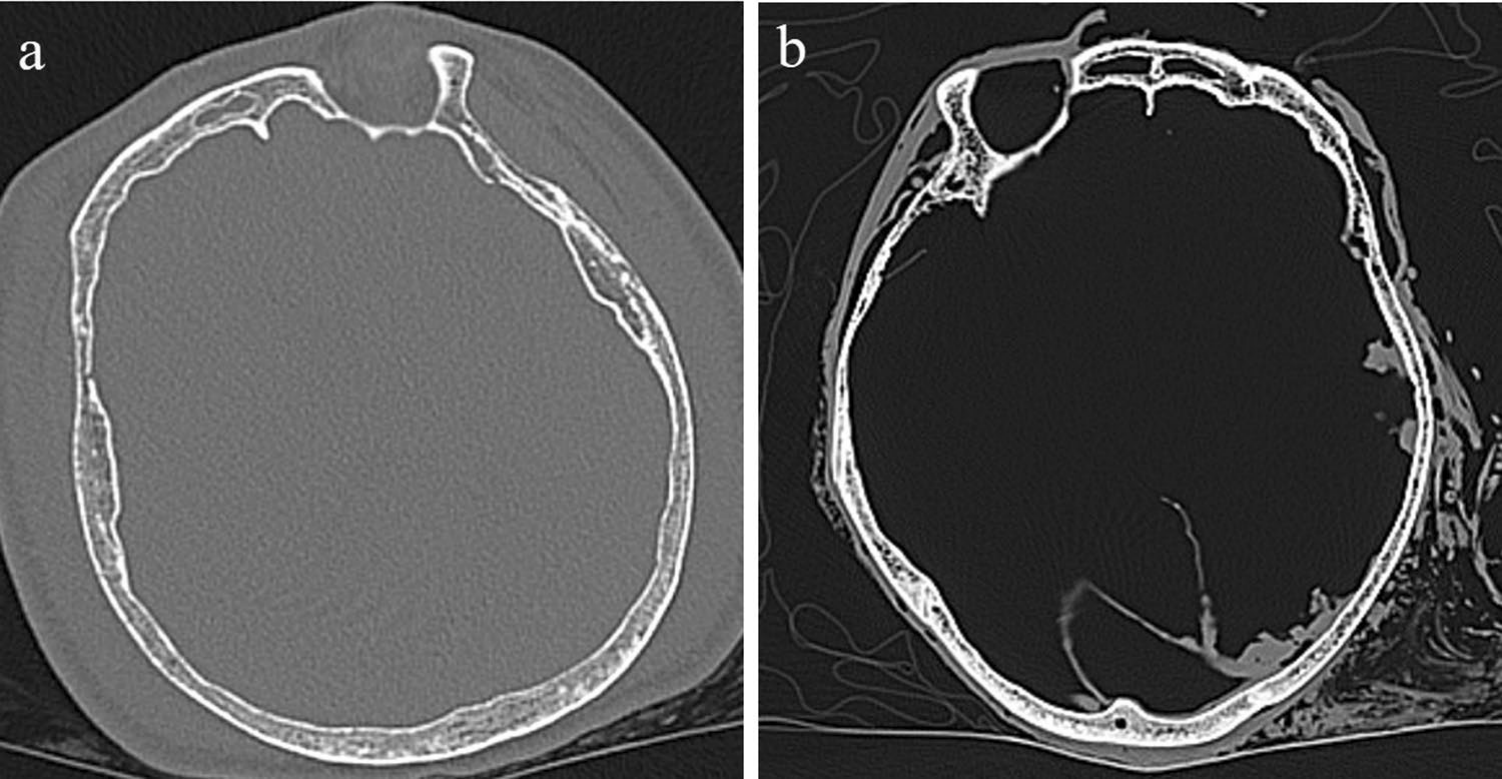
Comparison between the short and long postmortem intervals. (a) The CT slice within 12 h from the time of death. (b) The CT slice more than 1 month after the time of death. The putrefied gas can be confirmed in the cancellous bone of the skull.
